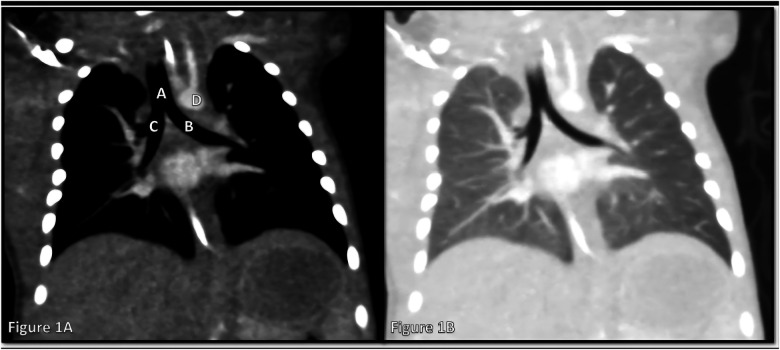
Coronal chest CT images demonstrating normal central airway anatomy and vascular relationships. (A) Lung window view showing the trachea (A), right main bronchus (B), left main bronchus (C), and the aortic arch (D) coursing over the left main bronchus without evidence of airway compression. (B) Corresponding coronal reconstruction highlighting normal lung parenchyma and airway branching.
